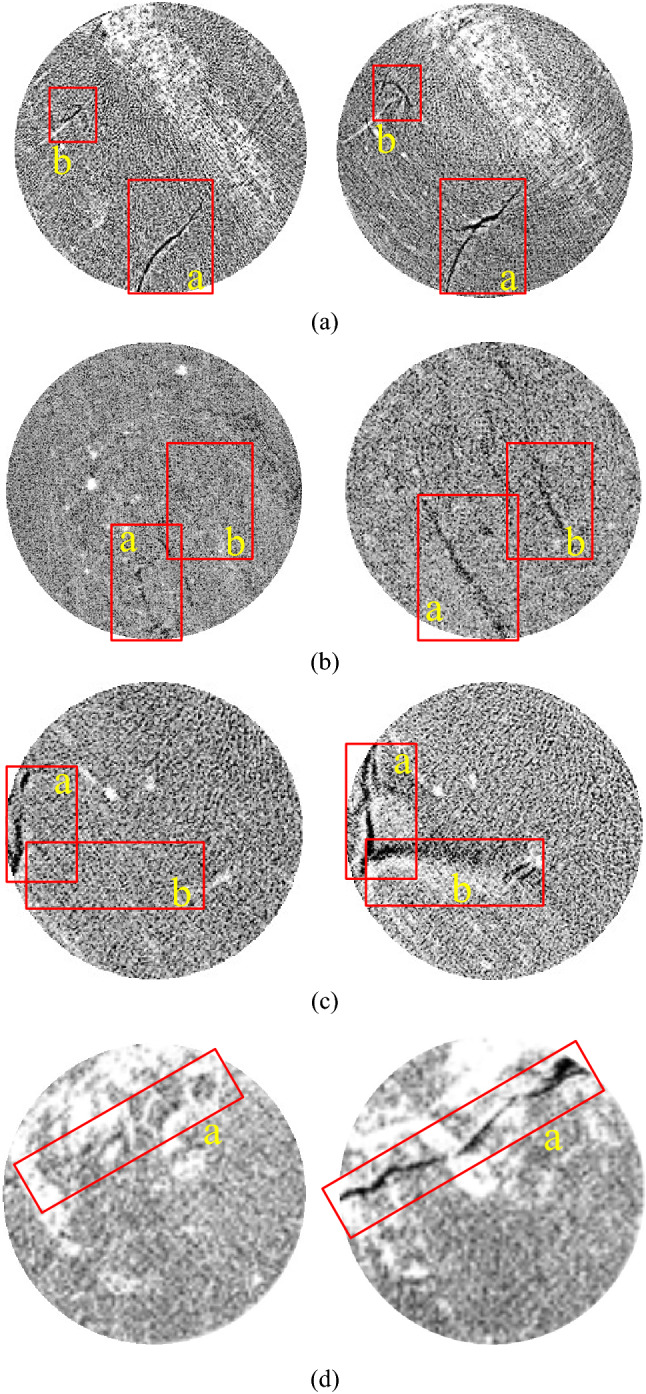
CT observation of coal sections before and after ultrasonic irradiating. (a) Sample ^#^4 at No.5 section, (b) Sample ^#^5 at No.3 section, (c) Sample ^#^6 at No.6 section, (d) Sample ^#^10 at No.1 section.
